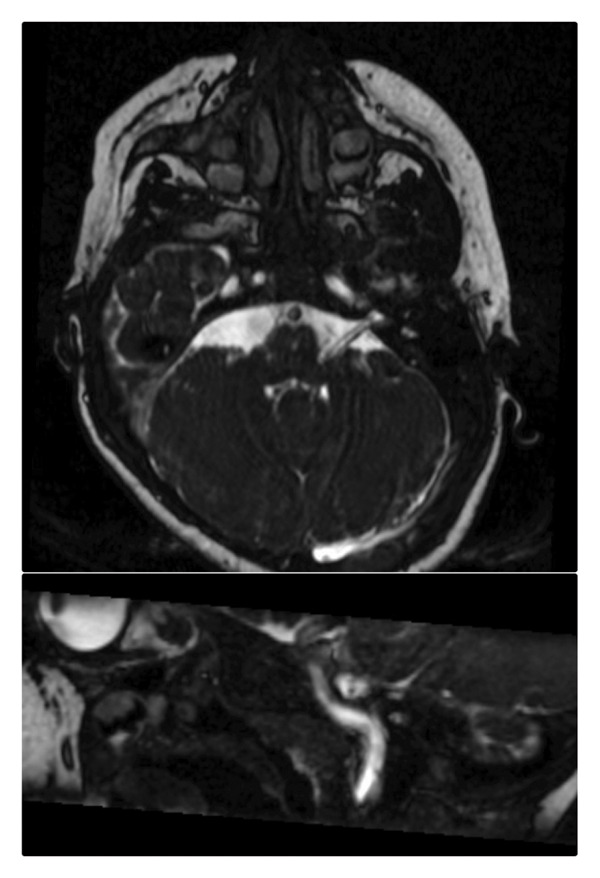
High‐resolution magnetic resonance imaging (MRI) of the temporal bones demonstrating the abnormal inner ear anatomy associated with bilateral common cavity deformities. Both superior semicircular canals and the left posterior semicircular canal are identifiable. MRI was primarily used to assess the vestibulocochlear nerves, which were bilaterally identifiable although reduced in caliber.
